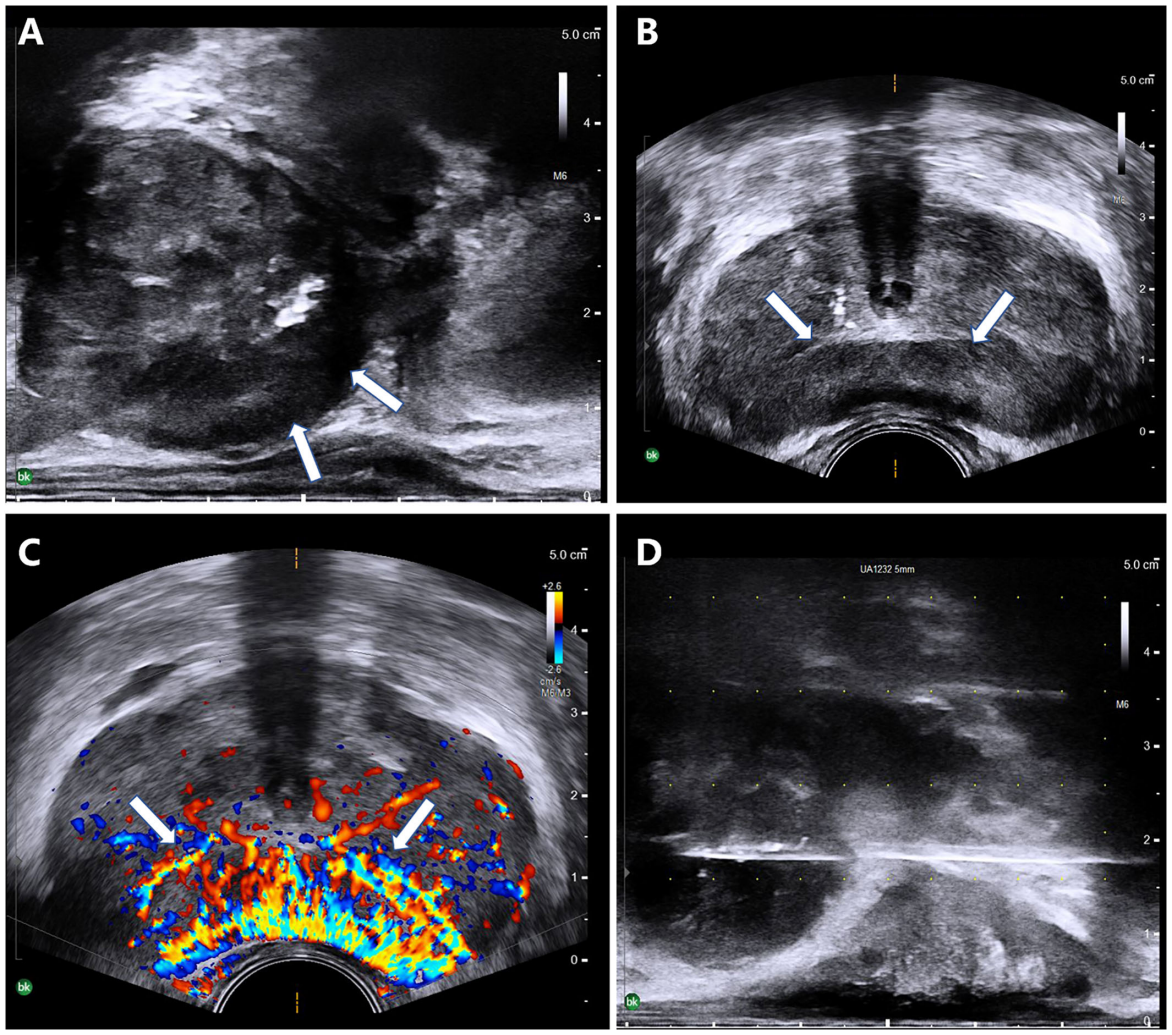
A 68-year-old man with elevated prostate-specific antigen (PSA). (A–D) Ultrasound revealed a morphologically abnormal prostate (A) and a hypoechoic nodule (*arrows*) at 3–8 o’clock in the peripheral zone (B), with an abundant blood flow signal in it (C). (D) A transperineal prostate biopsy was performed under ultrasound guidance.
